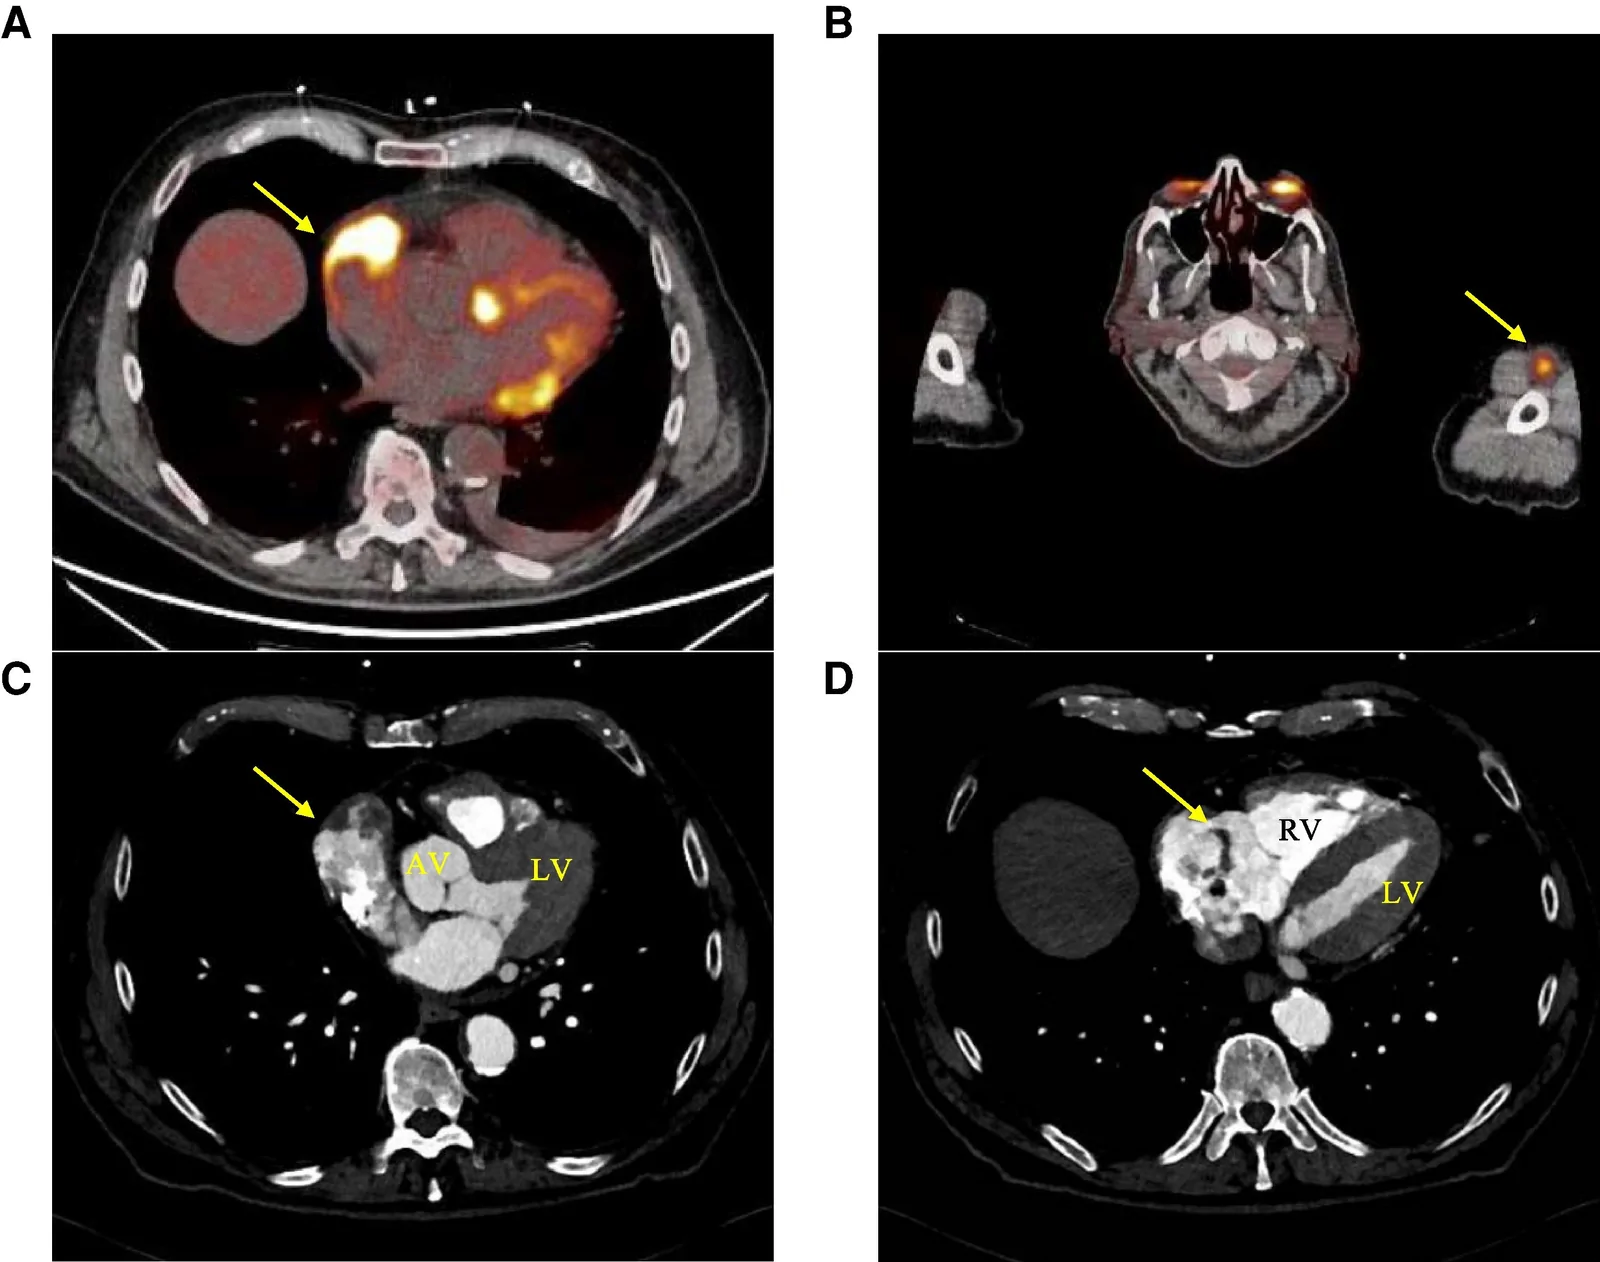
Advanced imaging at tertiary centre. (*A*) Positron emission tomography-computed tomography (PET-CT) demonstrating intense FDG uptake in the cardiac mass (arrow). (*B*) PET-CT showing an FDG-avid lesion in the left upper arm. (*C*) Photon-counting CT confirming mass origin from the right atrial appendage base with diffuse myocardial thickening (arrow). (*D*) Photon-counting CT demonstrating linear hypodense structure extending medially and dorsally from the right atrial appendage towards the inferior vena cava (arrow). AV, aortic valve; LV, left ventricle; RV, right ventricle.
